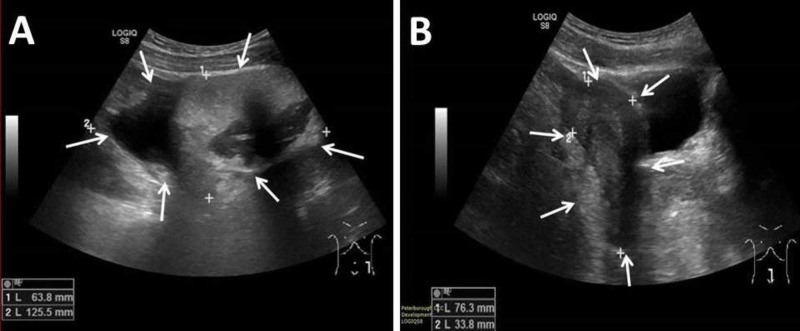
Ultrasound scan of the ovaries A. Left ovary demonstrating a large dermoid cyst, B. Right ovary demonstrating a large dermoid cyst
